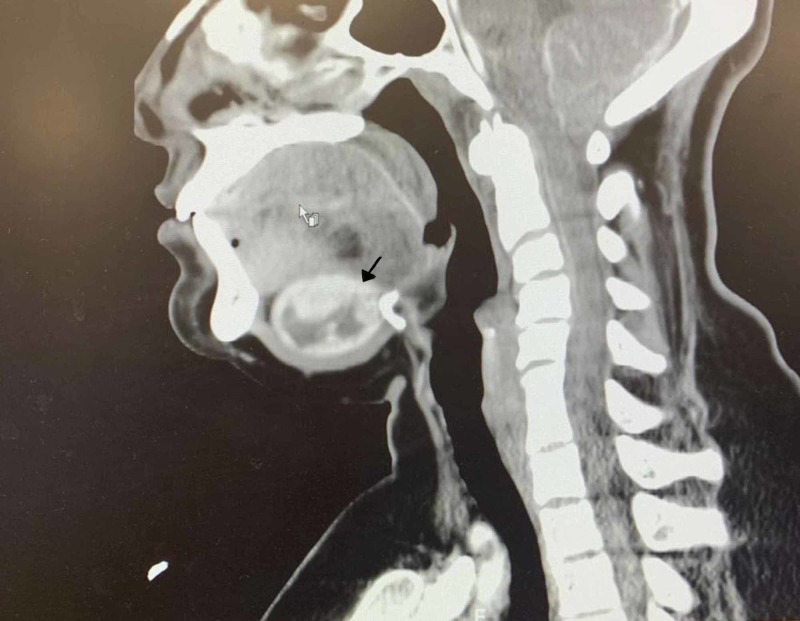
CT with contrast, lateral view demonstrating ectopic sub-lingual thyroid.
